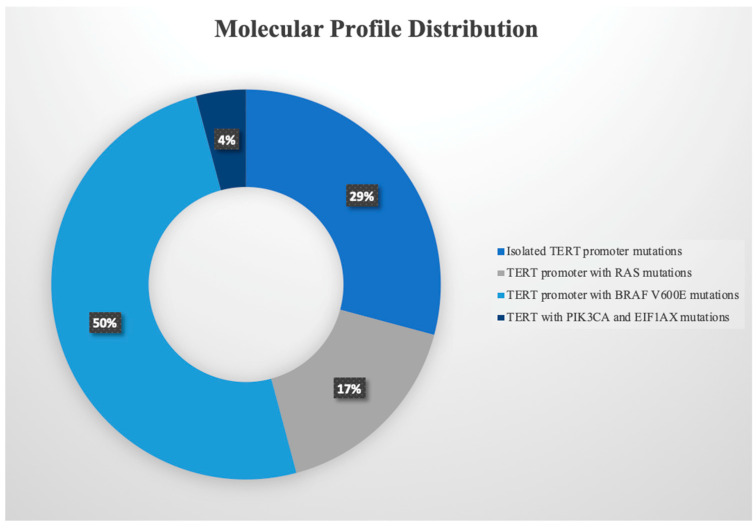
Mutation profile distribution of patients who underwent ultrasound-guided fine needle aspiration of a dominant thyroid nodule and tested positive for a *TERT* promoter mutation using ThyGeNEXT^®^ or Thyroseq V3^®^.
